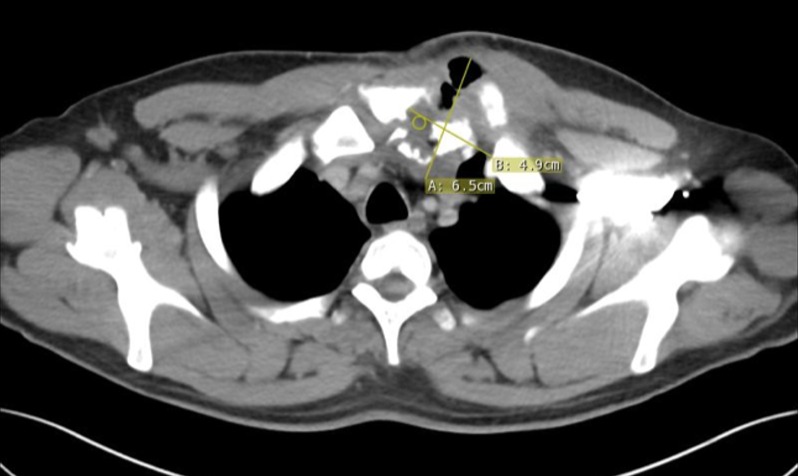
Axial CT Chest An axial CT of the chest with IV Contrast demonstrating destructive changes at the left SCJ and first costochondral junction with fluid collection and air measuring up to 6.5 cm consistent with septic joint and osteomyelitis.
